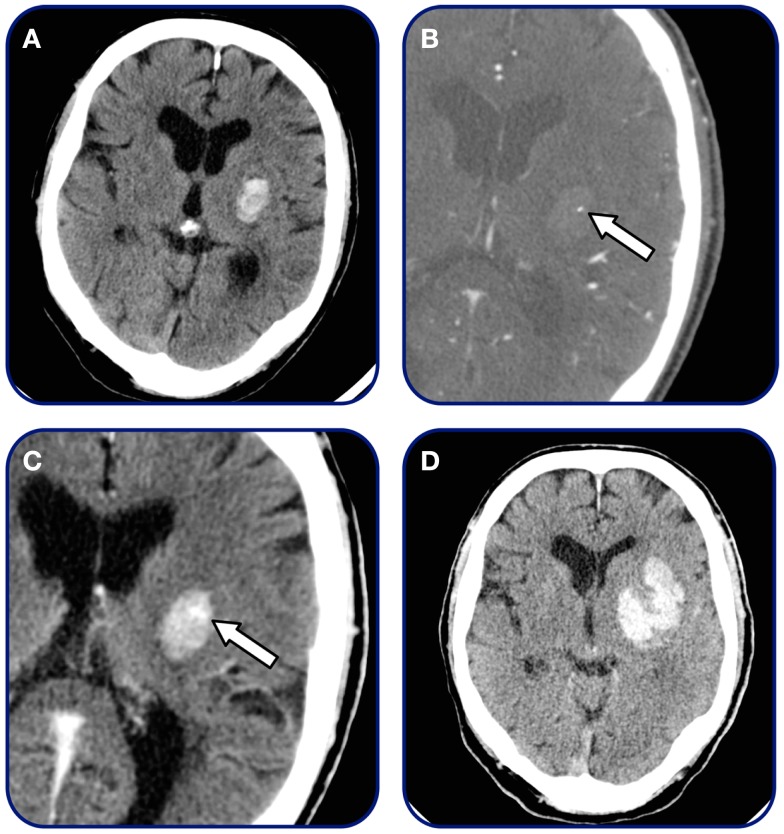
Spot sign and contrast extravasation. Patient admitted within 2 h after symptom onset. (A) Initial non-contrast CT demonstrating small basal-ganglia hemorrhage, (B) CTA source image demonstrating single spot sign (arrow), (C) 3-min post-contrast CT-imaging demonstrating contrast extravasation (arrow), and (D) 24 h follow-up CT revealing final hematoma volume.
